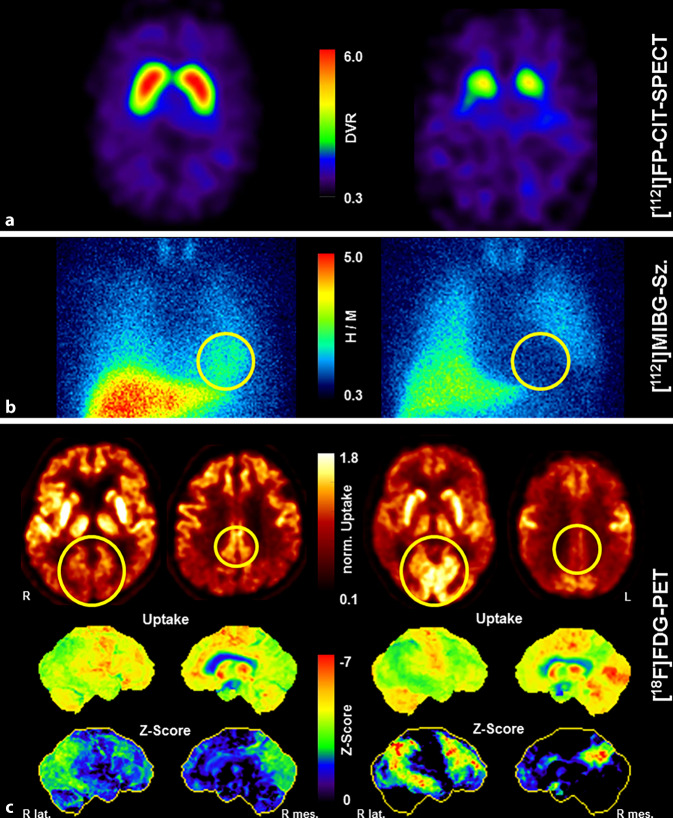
Das radioaktiv markierte Noradrenalinanalog [^123^I]MIBG wird zur szintigraphischen Darstellung der postganglionären sympathischen Innervation des Herzens eingesetzt, welche bei DLK und PK im Gegensatz zur AD und den sog. atypischen Parkinson-Syndromen (APS) oft bereits im frühen Krankheitsstadium reduziert ist (Abb. 1b). In einer frühen Metaanalyse wurde eine sehr hohe Sensitivität und Spezifität dieser Methode (98 % bzw. 94 %) zur Abgrenzung der DLK gegenüber anderen demenziellen Erkrankungen beschrieben [42]. Es ist jedoch darauf hinzuweisen, dass hier die klinische Diagnose nach damaligen DLK-Kriterien als Referenz diente, die selbst nur eine eingeschränkte diagnostische Genauigkeit besitzt (ca. 80 % [43]). In einer jüngeren, größeren japanischen Multicenterstudie mit 3‑jährigem Follow-up wurde die Sensitivität und Spezifität der [^123^I]MIBG-Szintigraphie mit 77 % bzw. 97 % für die Differenzierung zwischen wahrscheinlicher DLK und AD angegeben (Fälle mit möglicher DLK wurden ausgeschlossen) [44]. Bisher gibt es nur wenige Daten zur notwendigen Validierung der [^123^I]MIBG-Szintigraphie gegenüber der Post-mortem-Neuropathologie, die jedoch eine sehr hohe Sensitivität (> 90 %) der [^123^I]MIBG-Szintigraphie nahelegen, auch wenn einzelne DLK-Patienten eine normale sympathische Innervation des Herzens zeigen können [45]. Wie die DAT-SPECT wird die [^123^I]MIBG-Szintigraphie daher in den neuen DLK-Kriterien auch als „indikativer Biomarker“ aufgeführt [1].
